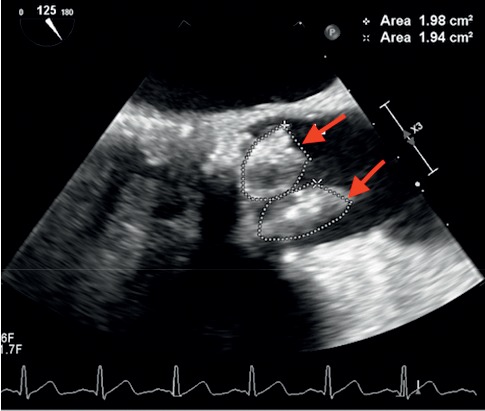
Transesophageal echocardiography showing two masses of approximately two cm^2^ each of mobile vegetations on the bioprosthetic aortic valve.
